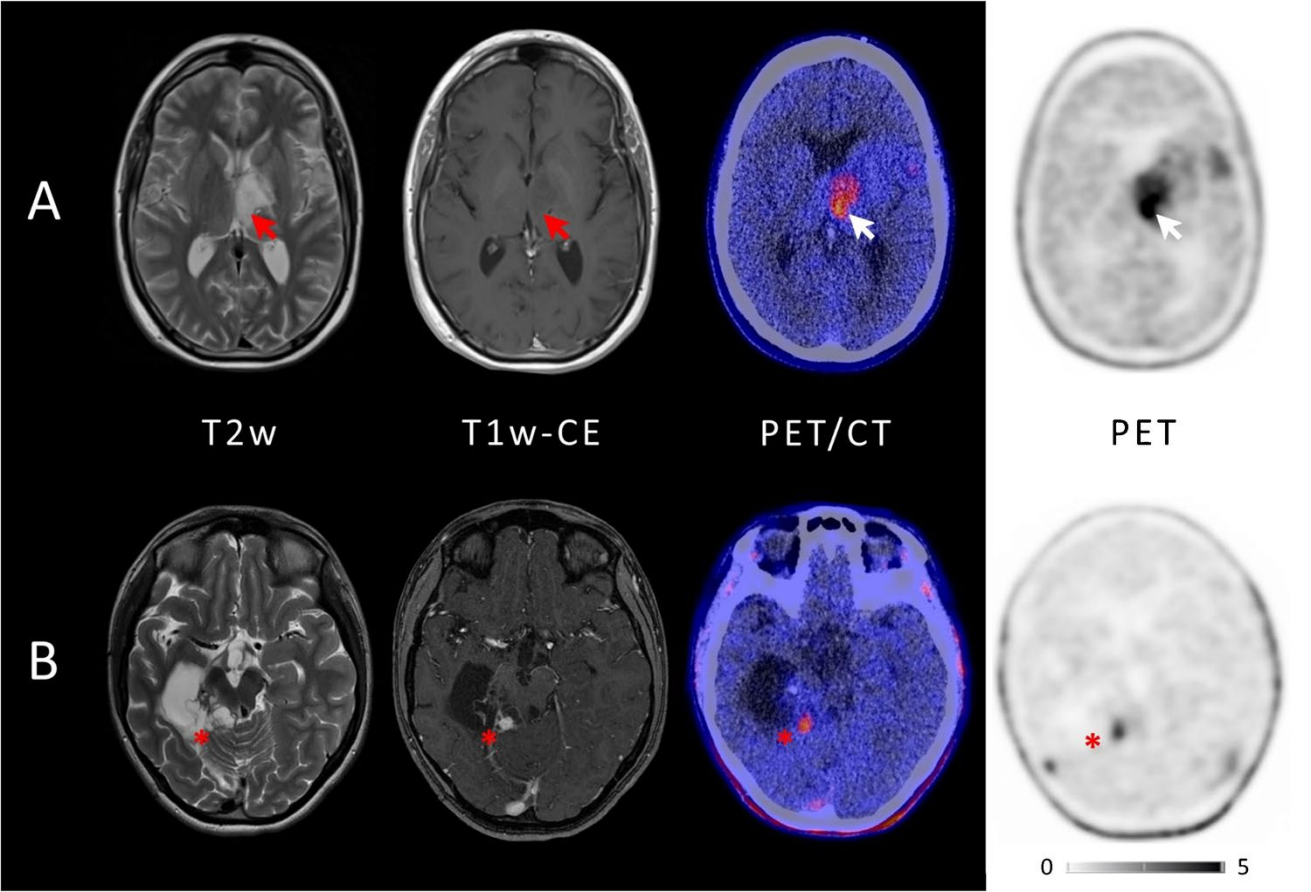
Example of two patients with concordant imaging results (A) and incremental diagnostic value of [^18^F]FET-PET (B). A Example of a patient (#10) with an anaplastic astrocytoma WHO grade III who was treated with combined radiochemotherapy prior to PET. Axial T2WI and T1WI + CE depict the tumor in the left thalamus and insular region (red arrows); axial PET shows high uptake of [^18^F] FET in these regions (white arrows). Both MRI and PET identified correctly the tumor recurrence. B Example of a patient (#19) with a pilocytic astrocytoma who was treated with surgery and chemotherapy prior to PET. Axial T2WI and T1WI + CE show cystic as well as contrast enhancing lesions next to the surgical cavity consistent with both unspecific changes and tumor recurrence (red asterisk); in contrast, axial PET demonstrates focal high uptake of [^18^F]FET (white asterisk)
